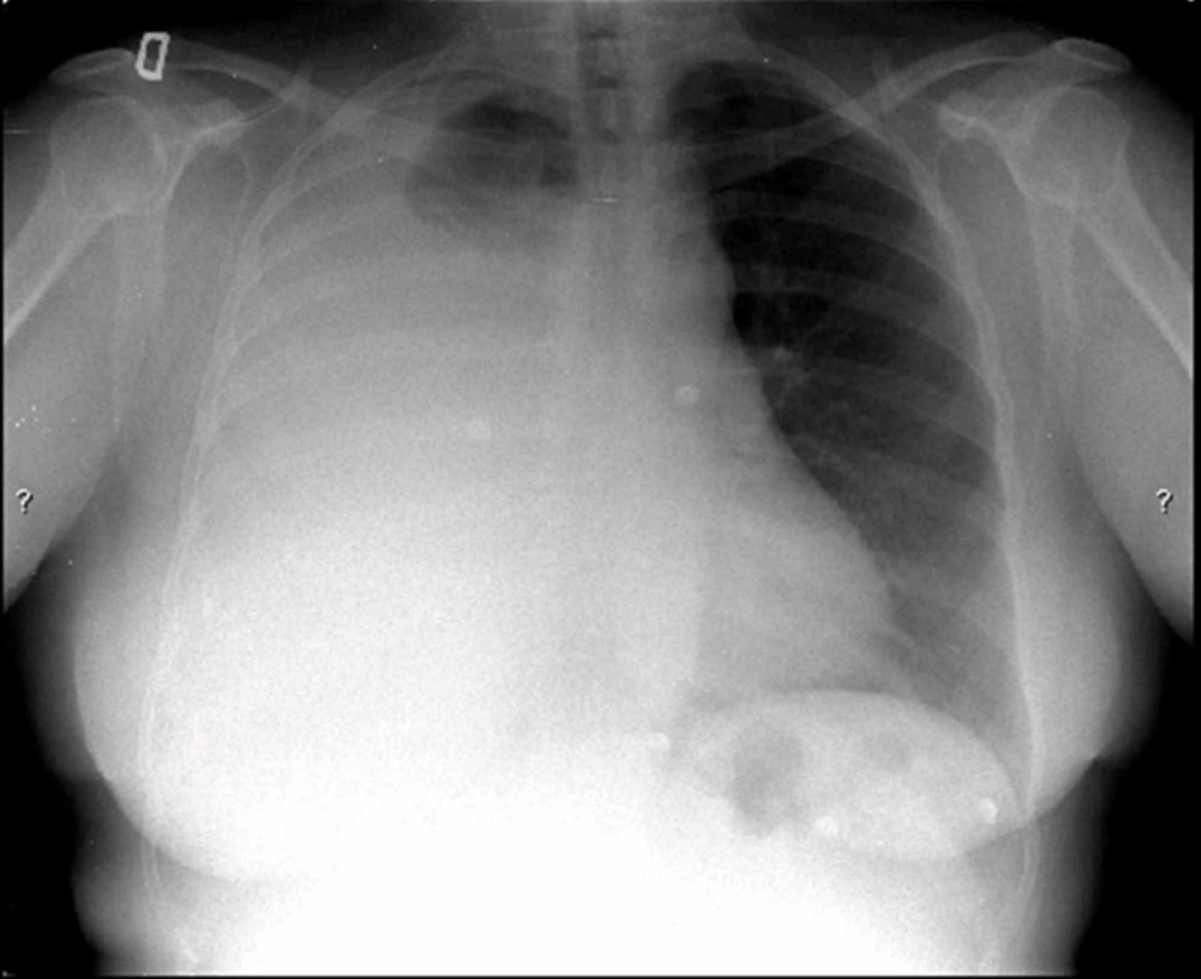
Chest X-ray on admission. A large right-sided pleural effusion is observed.
